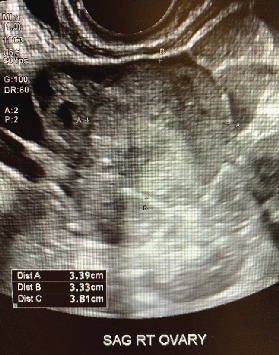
Sagittal view of right ovary on transvaginal ultrasound. The right ovary measures 5.24 cm × 5.52 cm × 3.22 cm. Within the right ovary is a solid heterogenous mass measuring 3.4 cm × 3.3 cm × 3.8 cm.
